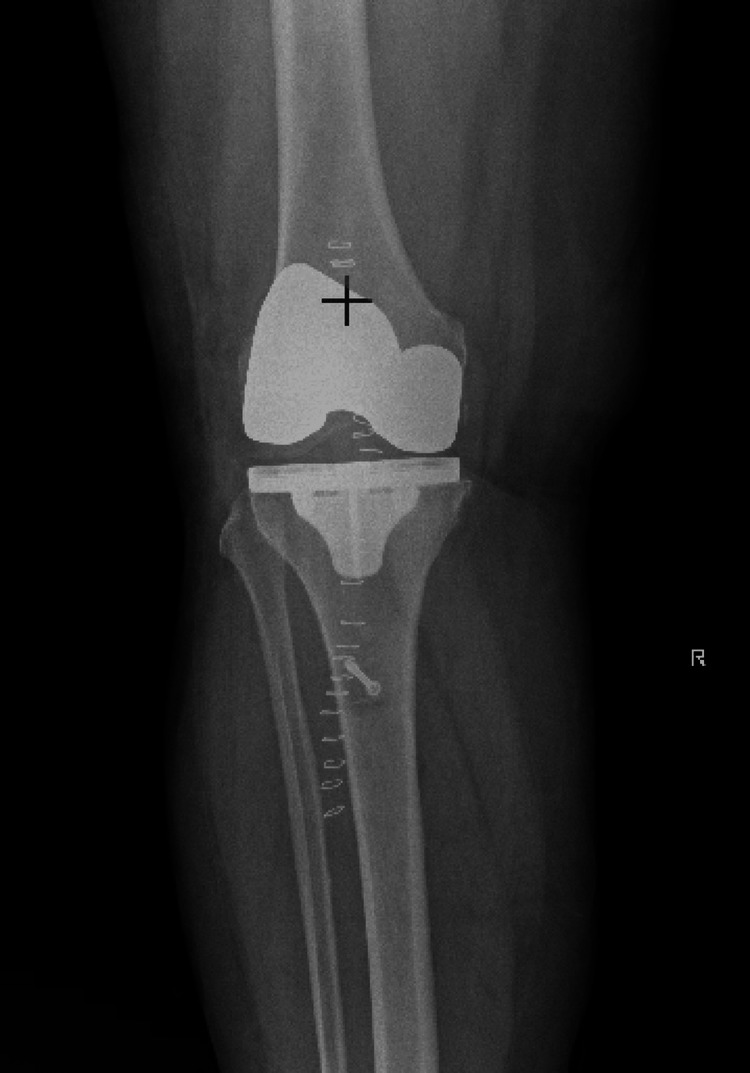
Postoperative anteroposterior radiograph of the right knee Postoperative anteroposterior radiograph of the right knee demonstrating intact total knee arthroplasty components with restored patellar height following Achilles tendon allograft reconstruction of the patellar tendon.
